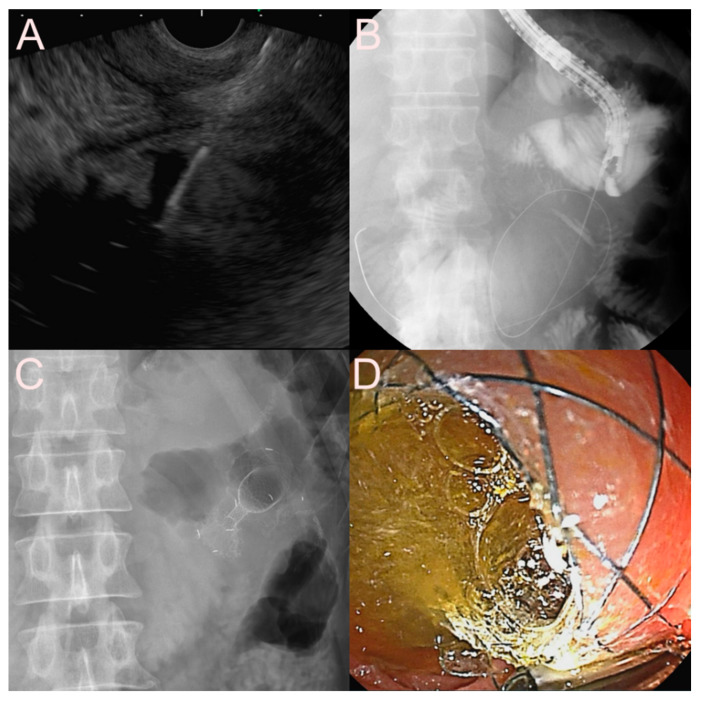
Ultrasound-guided jejunojejunostomy using the dedicated bi-flanged lumen-apposing metal stent (LAMS) (Niti-S SPAXUS; Taewoong Medical Co., Ltd., Ilsan, South Korea) for afferent loop syndrome in a patient who underwent total gastrectomy with Roux-en-Y anastomosis. (A) Endosonographic image showing a markedly dilated afferent loop with large amounts of sludge and heterogeneous debris. (B) Fluoroscopic image showing a guidewire delivered to the afferent jejunal loop through a 19-gauge needle punctured from the patent efferent jejunal loop. (C) Fluoroscopic image showing the dedicated bi-flanged LAMS placed between the afferent and efferent loops. (D) Endoscopic image showing the drainage of pus-like materials through the dedicated bi-flanged LAMS.
